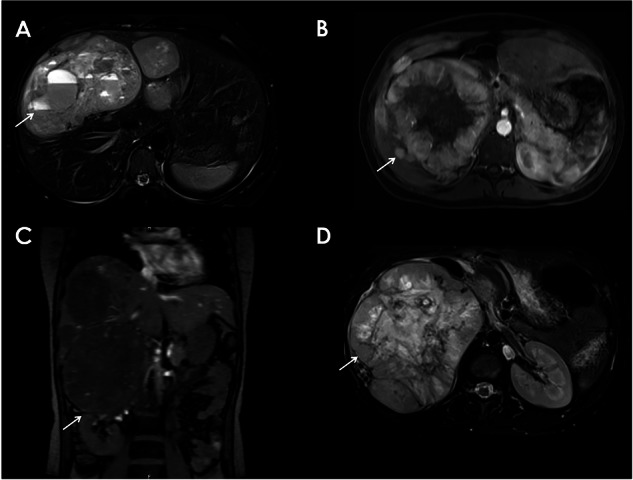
PCC with hepatic metastasis. Heterogeneous adrenal mass presenting a peripheral enhancement on T1-w CE MRI (B): central necrosis. We can see a downward displacement of the right kidney (C). Hepatic mass, with a cystic component hyperintense on T2-wMRI (A), with a heterogeneous enhancement on T1-w CE MRI (D)
